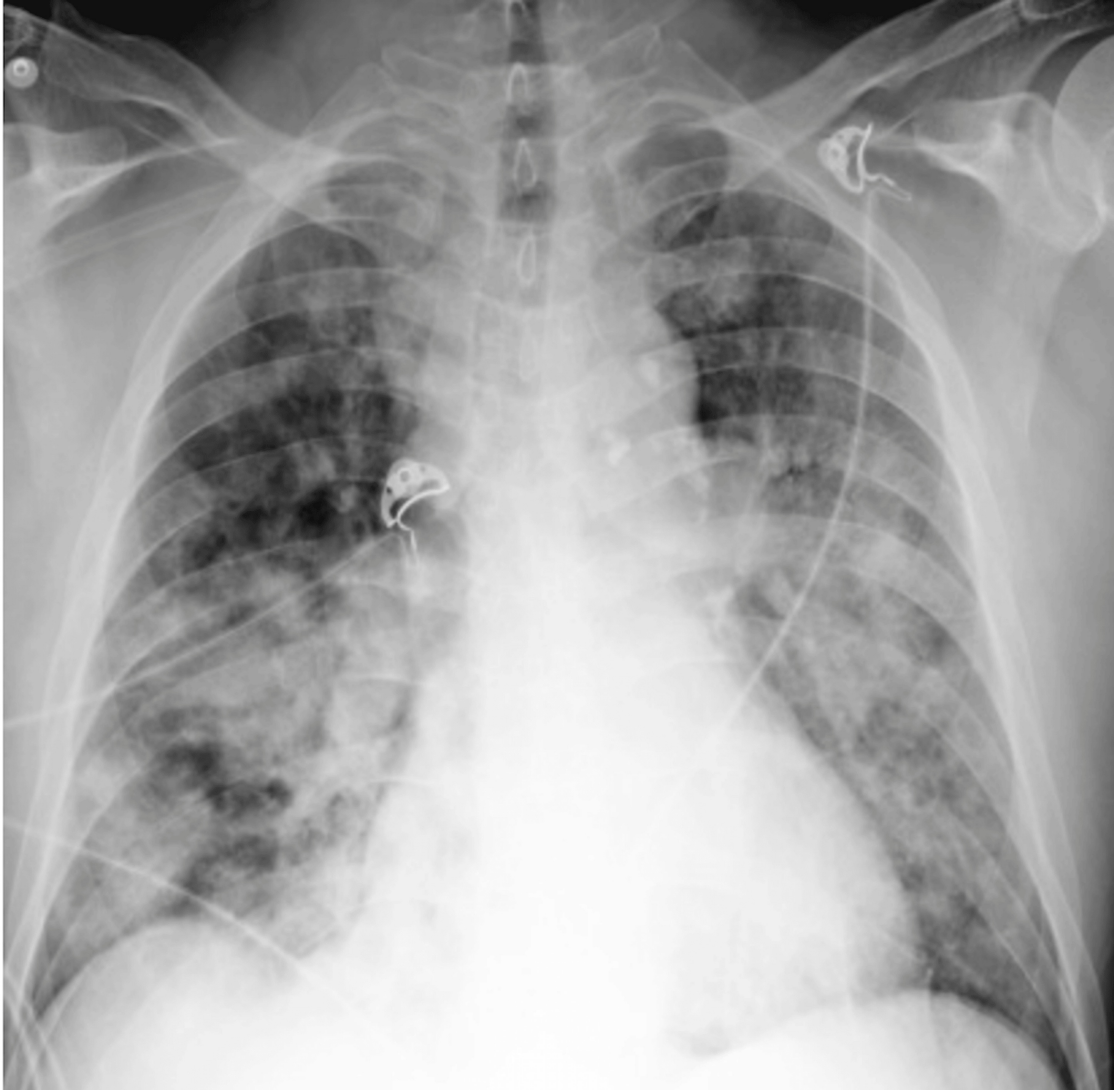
Chest X-ray on admission
